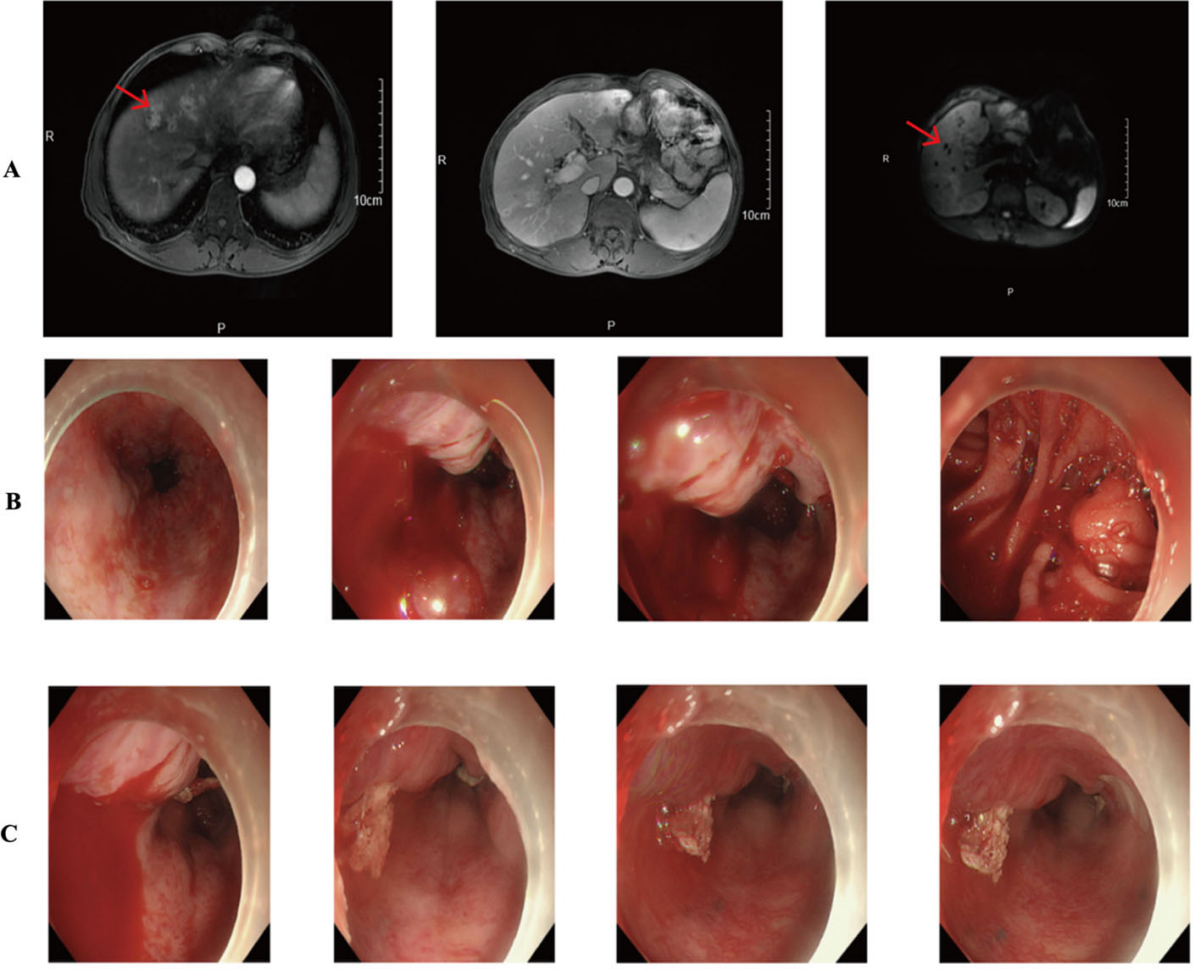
(A) CT results showed multiple intrahepatic metastases. (B, C) Gastroscopy suggests active gastrointestinal bleeding.
